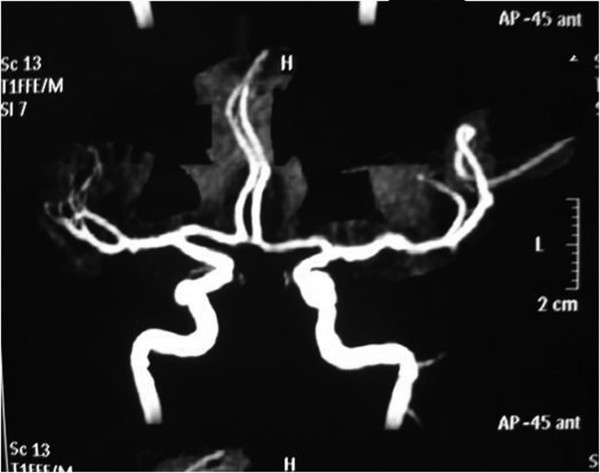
Brain MRI Angiography. Normal.
